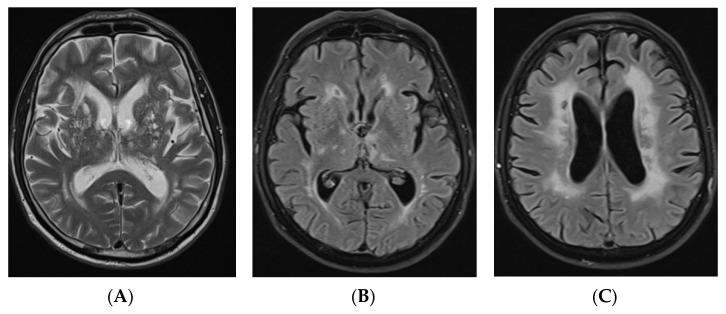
Axial T2-weighted image (A) and FLAIR axial images (B,C) of brain MRI demonstrating subcortical and periventricular T2/T2 dark-fluid hyperintensities, Fazekas scale score 2–3, lacunar lesions in the thalamus bilaterally, and hydrocephalus (HI—66). FLAIR: fluid attenuated inversion recovery; MRI: magnetic resonance imaging, HI: Huckman index.
